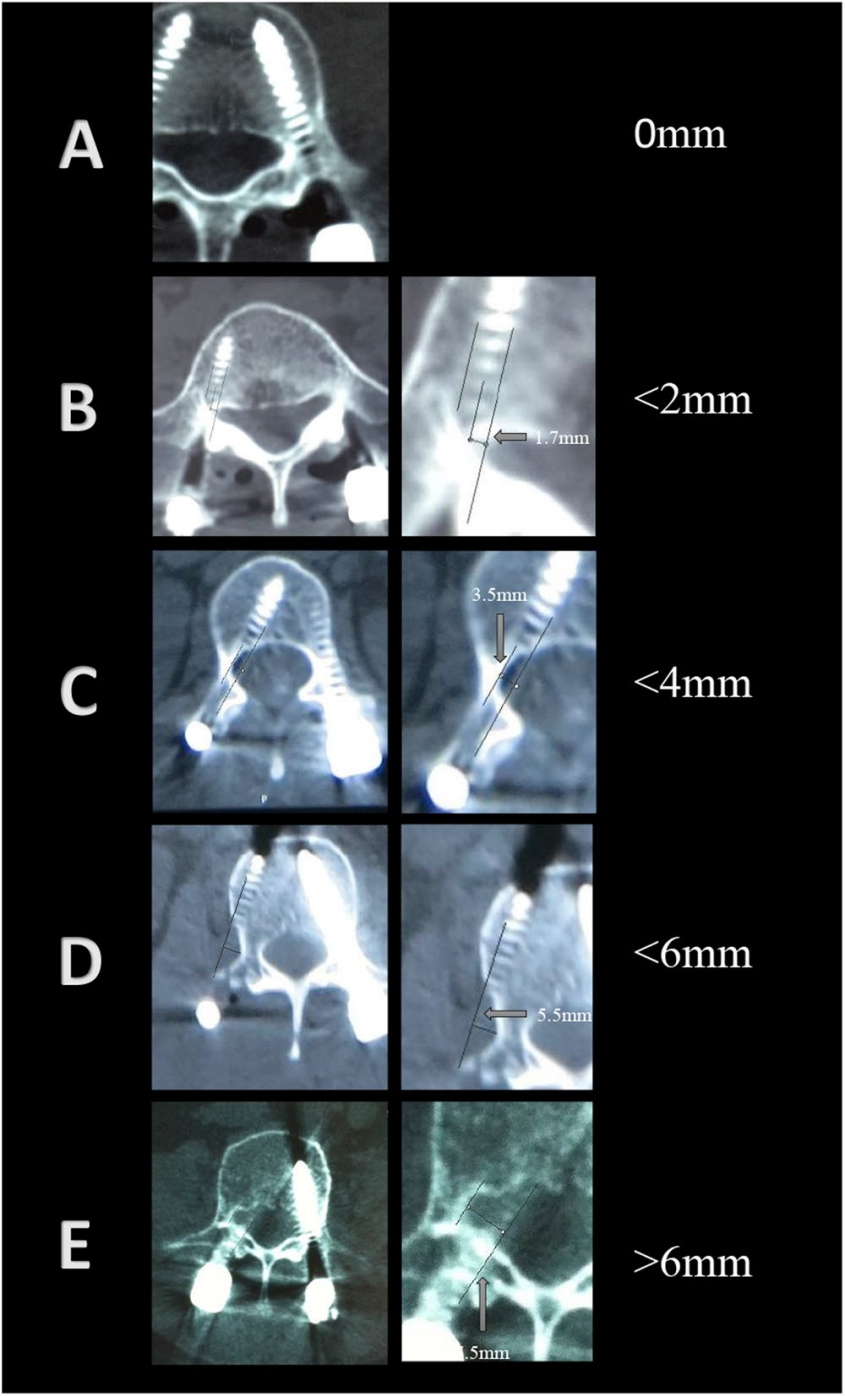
Gertzbein and Robbins classification scores are shown based on CT scans to reflect the deviation of the screw from the optimal trajectory. Grade (A): screw is completely within the pedicle; Grade (B), screw breaches the pedicle’s cortex by <2 mm; Grade (C), pedicle cortical breach <4 mm; Grade (D), pedicle cortical breach <6 mm; Grade (E), pedicle cortical breach >6 mm.
